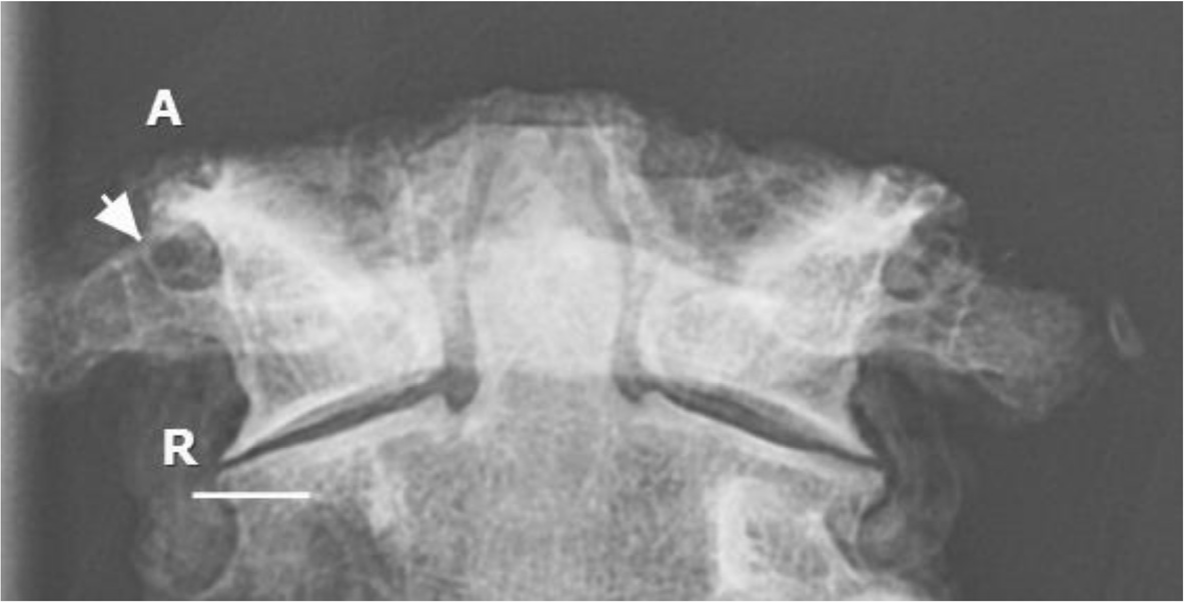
Frontal radiographic view of C1 and C2 vertebrae demonstrating complete lateral ponticle (*arrow*) on right and incomplete on the left. Linear bar represent 10 mm
